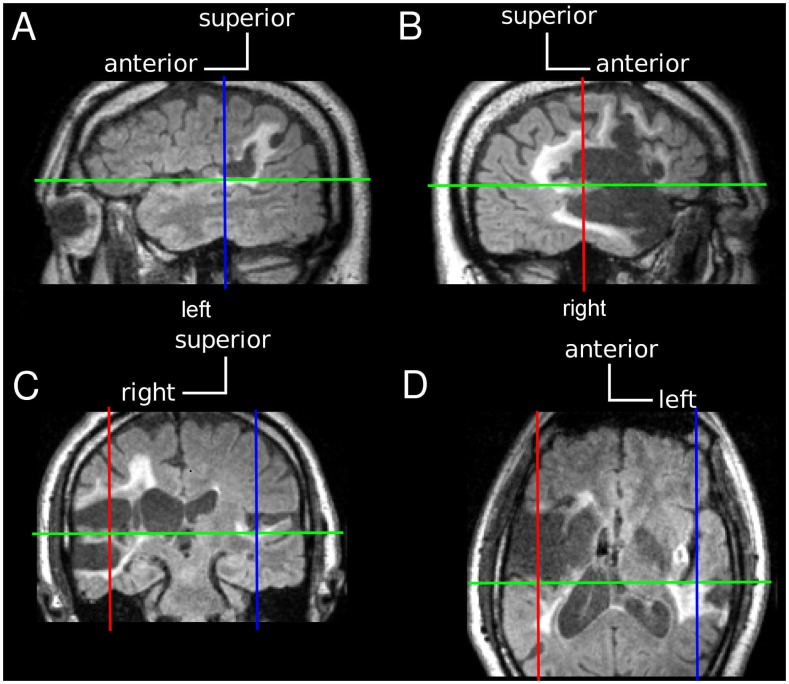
Case A1+ MRI FLAIR sequences. (A,B) Parasagittal sections through the left and right hemispheres. Left TG is atrophic and right TG is replaced by encephalomalacia (low signal intensity). Ischemic demyelination and retrograde degeneration within adjacent white matter regions appear as areas of high signal intensity. (C) Coronal section through the mid-portion of left and right TG and STG. (D) Horizontal section through left and right TG and STG. See text for image acquisition parameters.
